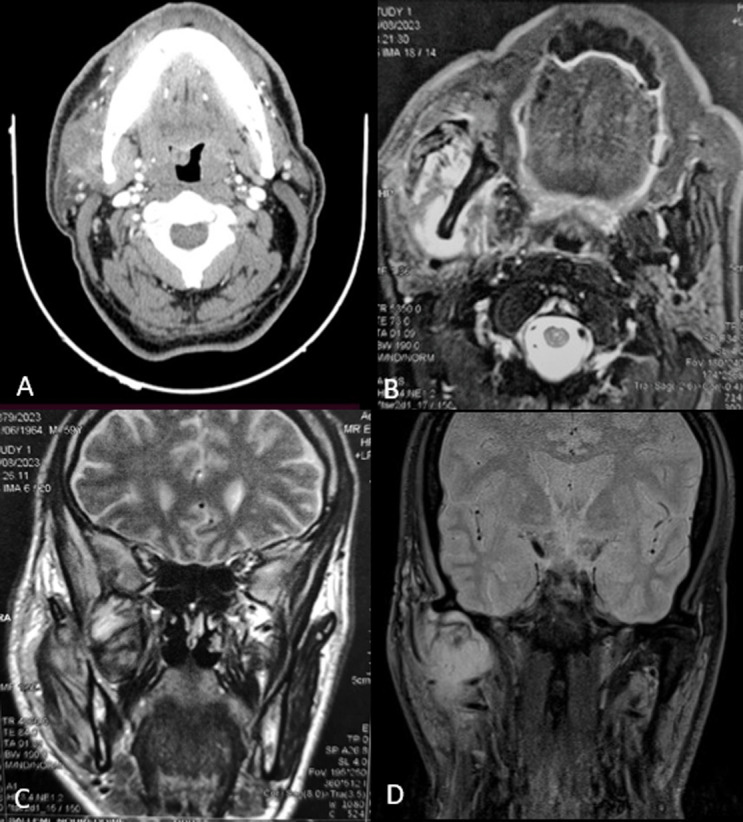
A) contrast-enhanced CT showing a cystic formation within the masticatory space centered on the lateral pterygoid muscle and the temporal muscle, with lateral extension encompassing the masseter muscle; B, C, D) MRI showing an expansive lesion process developing in the right masticatory space, with extended heterogeneous signal in the masseter, temporal, and lateral pterygoid muscles, including internally enhanced necrotic zones, also extending to the pterygo-palatine process
